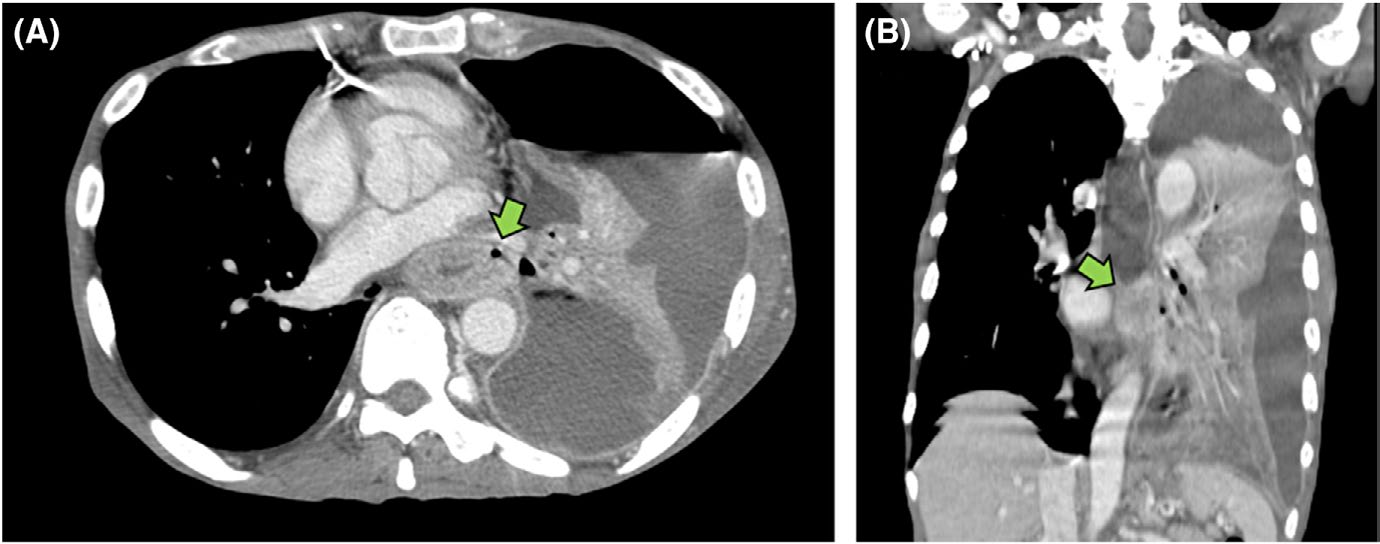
The same contrast‐enhanced CT images revealed circumferential thickening of the esophageal wall and intramural free air (A and B, arrow), suggesting esophageal cancer complicated by esophagorespiratory fistula.
